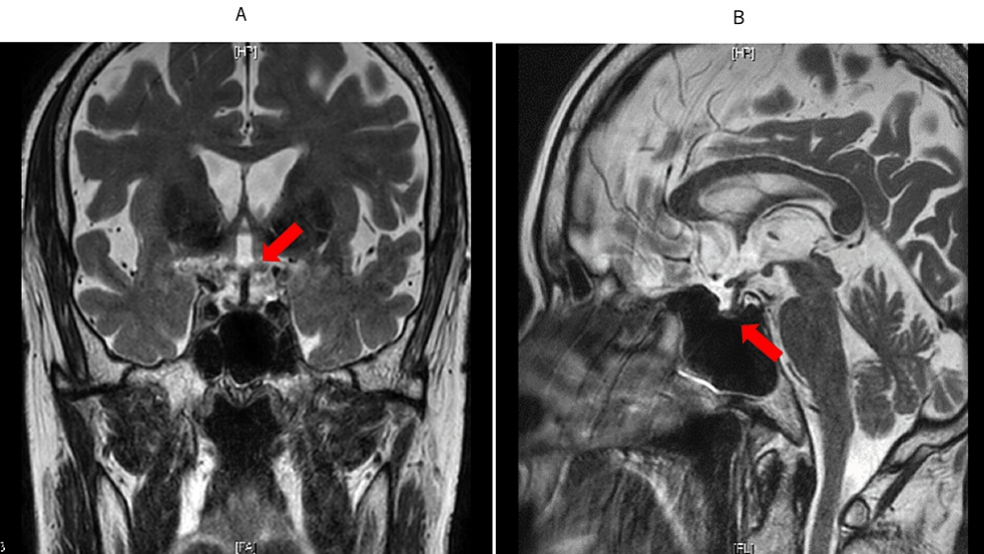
Brain MRI on June 2. A: Coronal section. B: Sagittal section. Note that the pituitary gland and pituitary pattern (arrows) were almost normalized.
